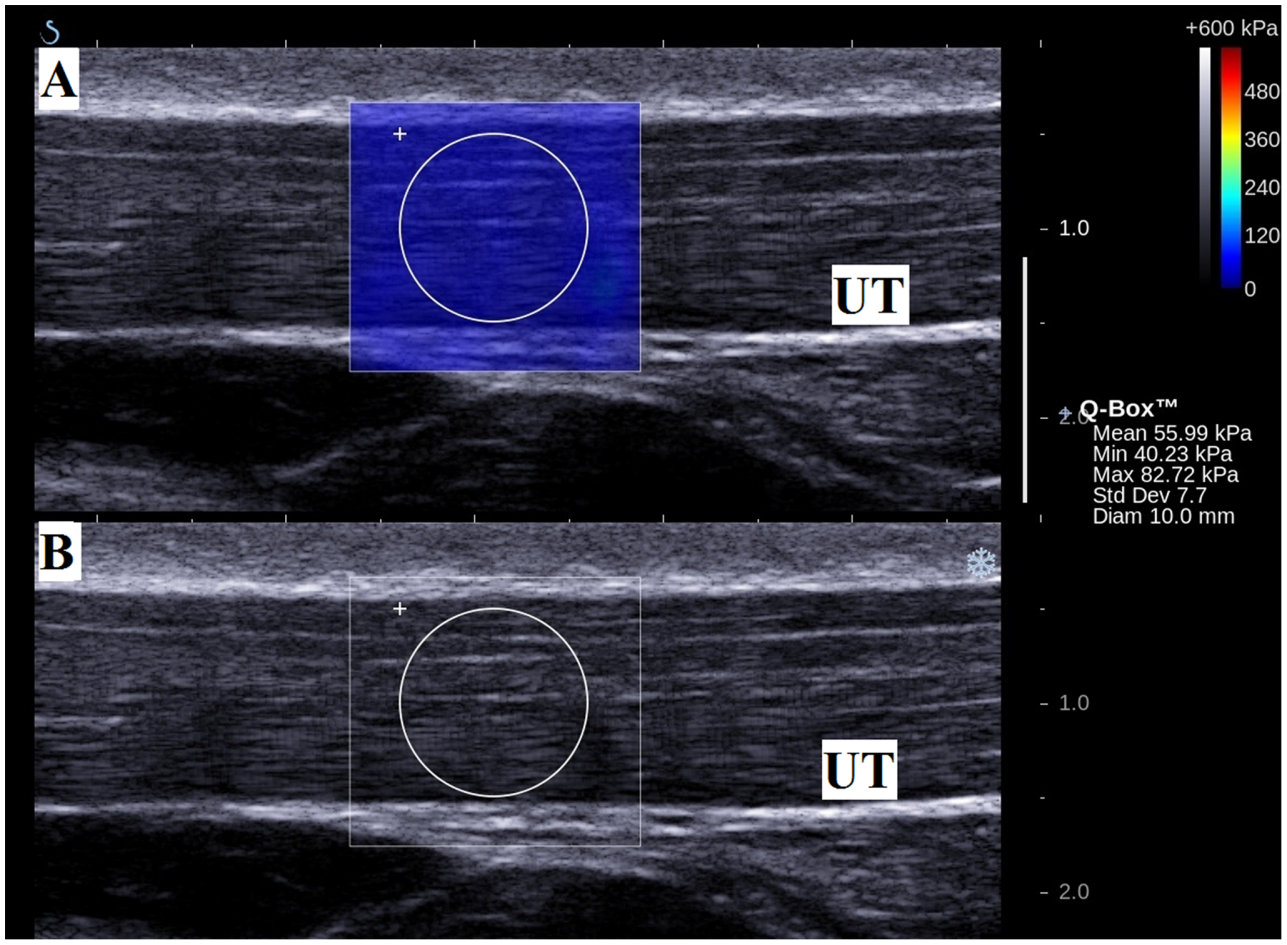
Measurement of elasticity of the upper trapezius muscle (UT) in longitudinal view. (A) Color-coded box presentations of the UT elasticity (stiffer areas were coded in red and softer areas in blue) superimposed on a longitudinal grey scale sonogram of the UT, with the circle representing the region of interest (ROI), and were adjusted according to the thickness of the muscle. The shear elastic modulus of the ROI is shown in the Q-box™ on the image. (B) Longitudinal grey scale sonograms of UT on the identical scan planes.
